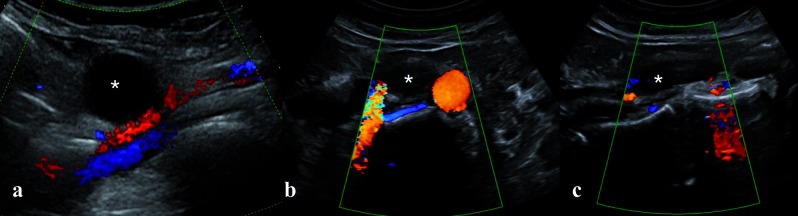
Color Doppler images of pelvic (a) and lomboaortic (b, c) lymphoceles (white asterisks) after systematic lymphadenectomy
